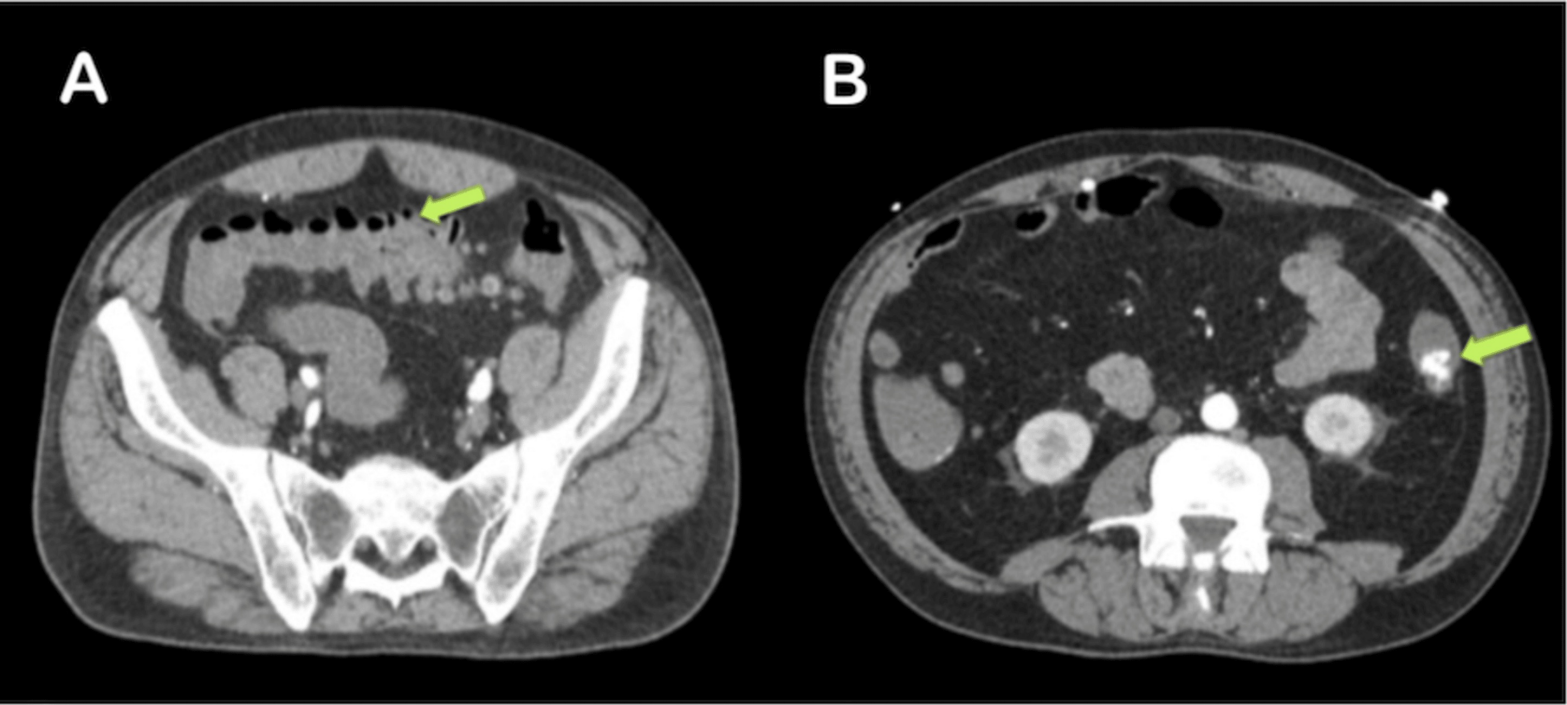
Abdominal CT with contrast demonstrating (A) diverticulosis without signs of diverticulitis and no evidence of bowel obstruction and (B) contrast extravasation within the lumen of the descending colon, indicative of a lower gastrointestinal bleed
